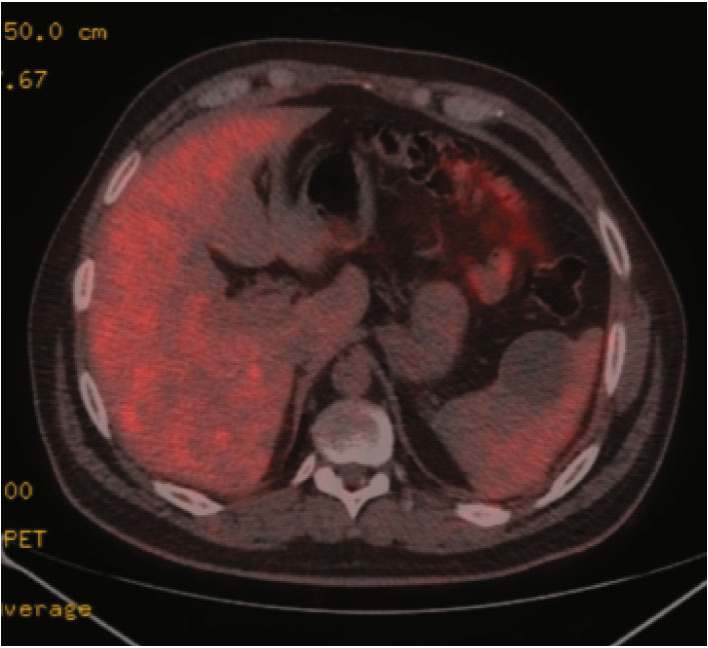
PET/CT images show a 40 mm lesion without increased metabolic activity.
